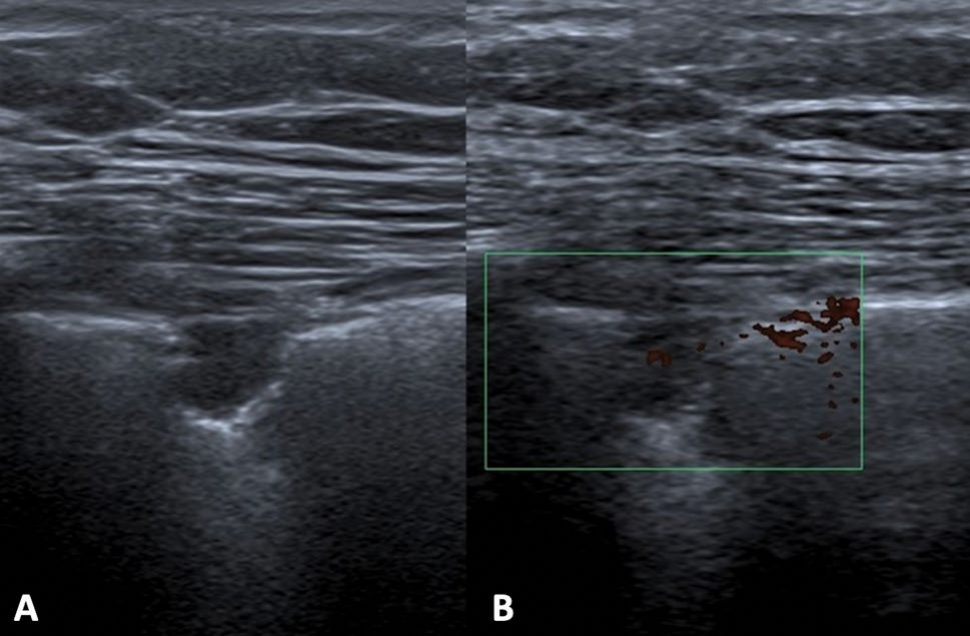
Female patient of 44 years old. B-mode (A) and power Doppler (B) scans. US shows a pleural metastasis as hypoechoic nodule, close to pleural line, characterized by irregular margins, posterior enhancement and intralesional vascularization on power Doppler
